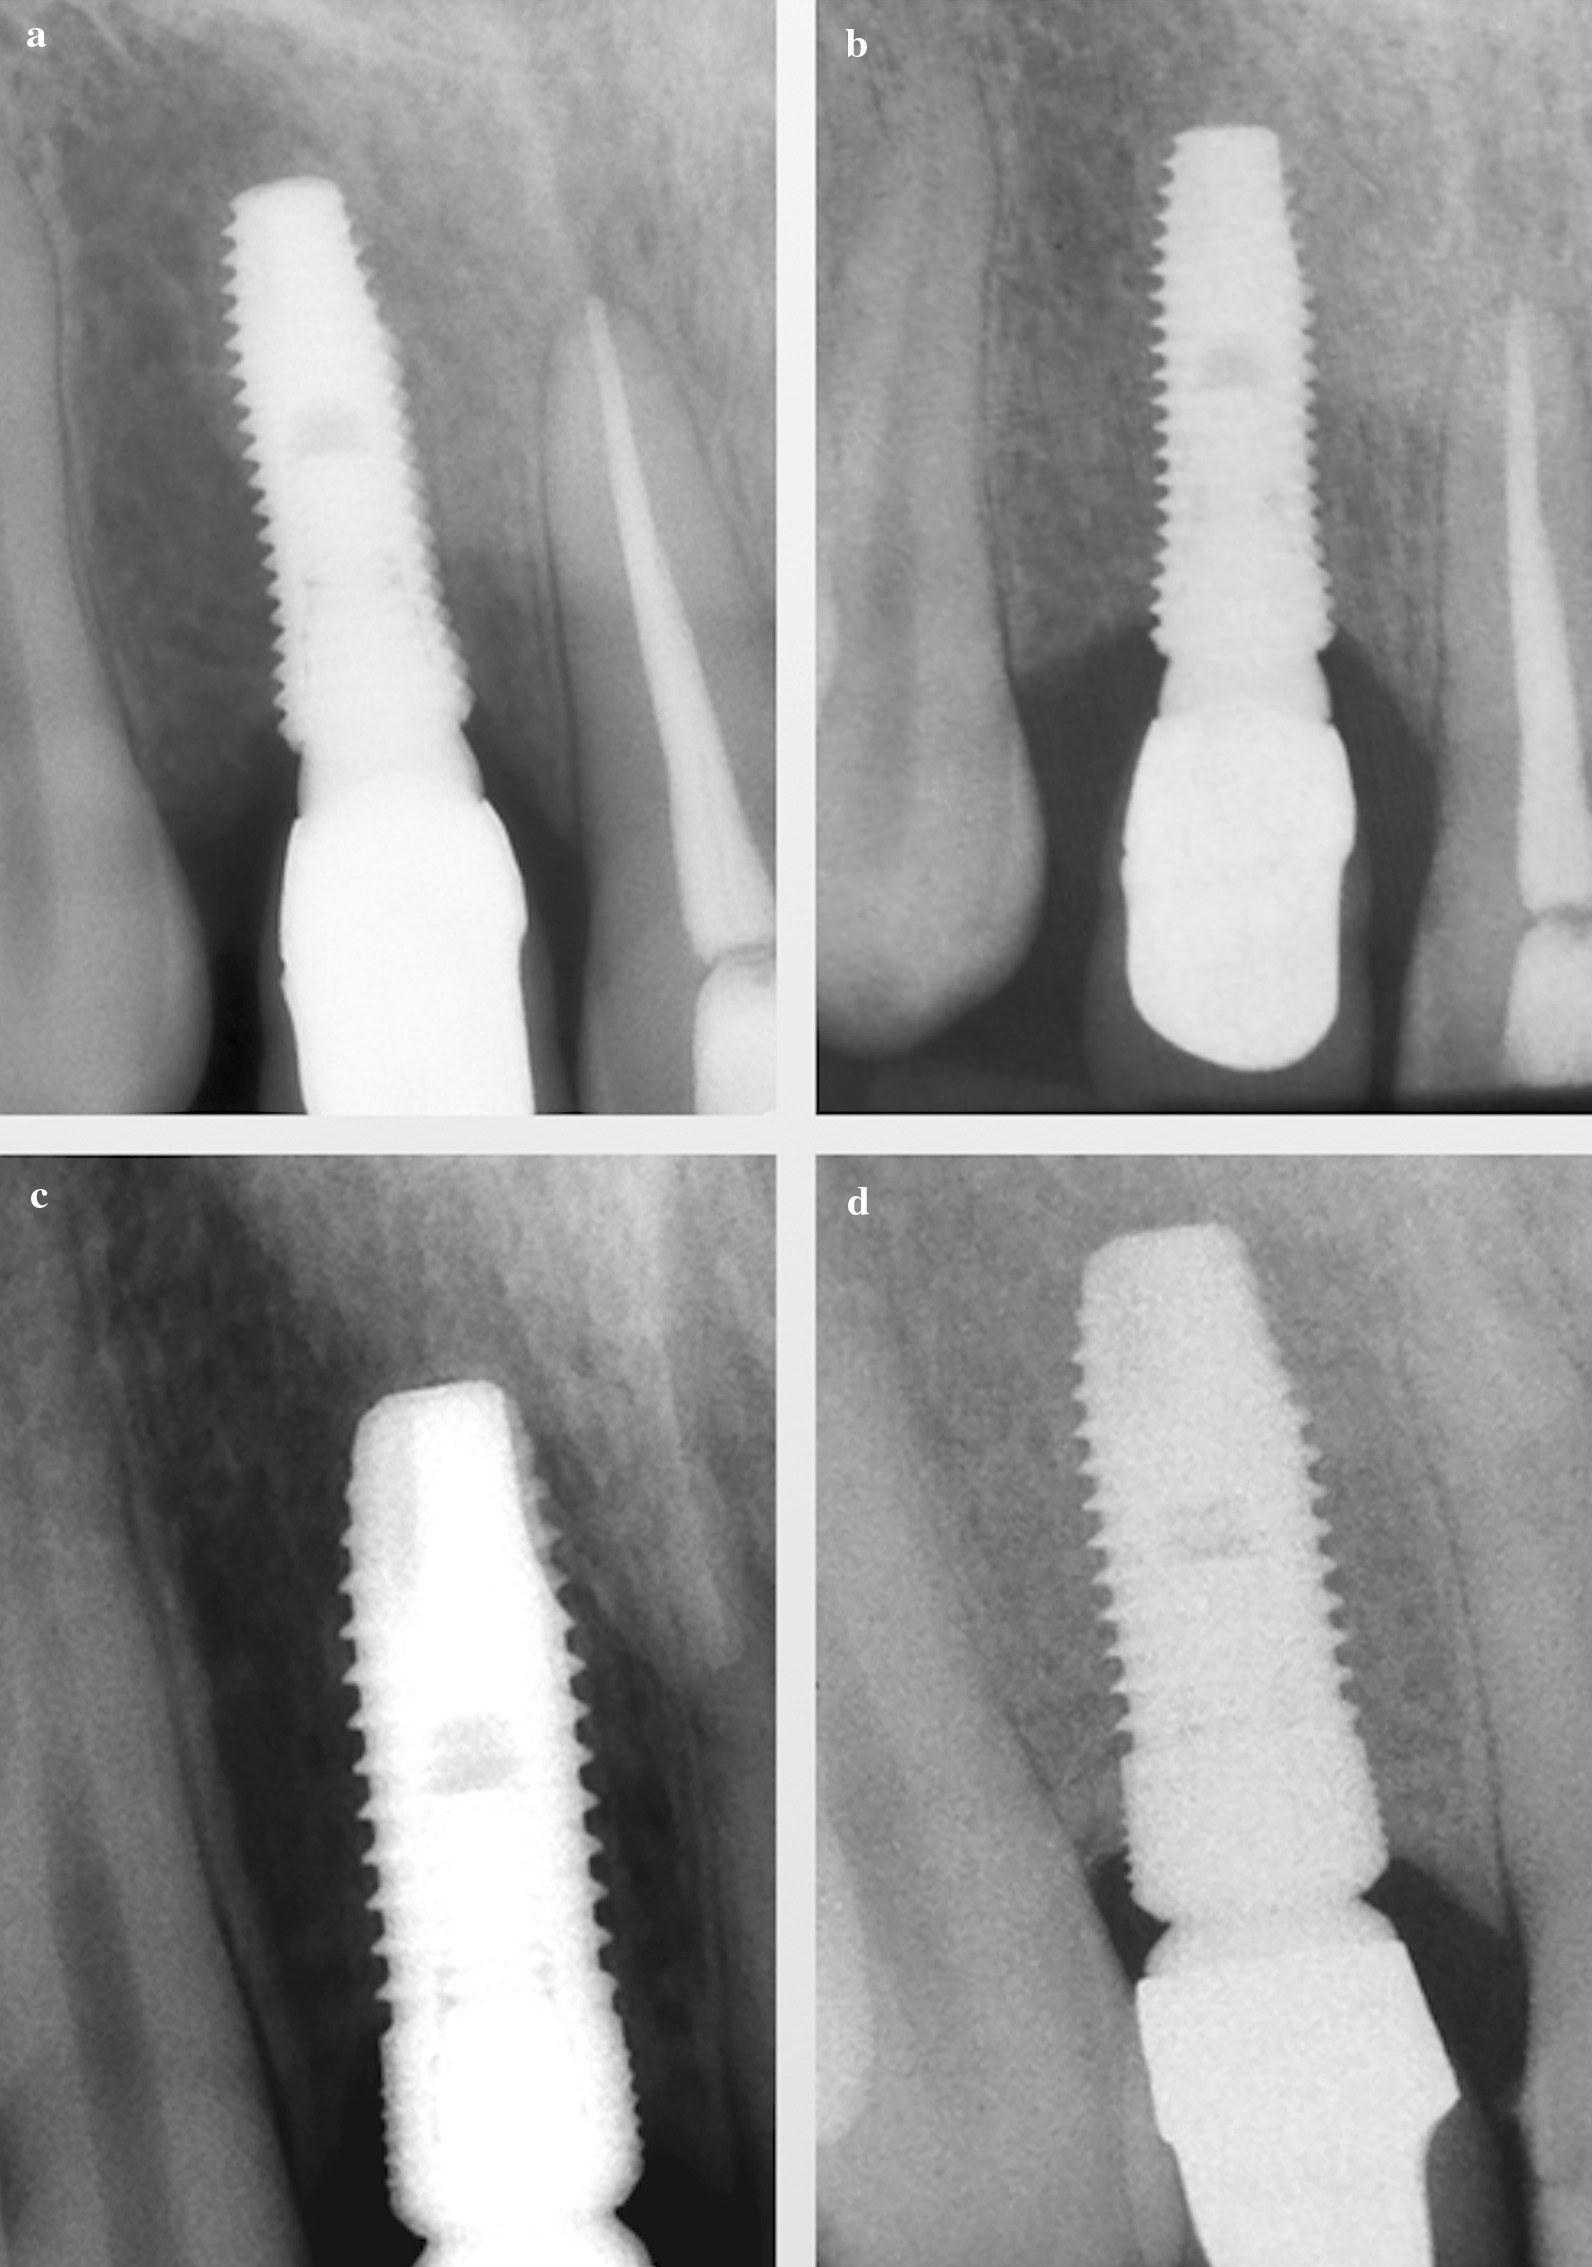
Intraoral periapical radiographs in treaded implant (a 1-year and b 5-year visit); and microthreaded implant (c 1-year and d 5-year visit)
